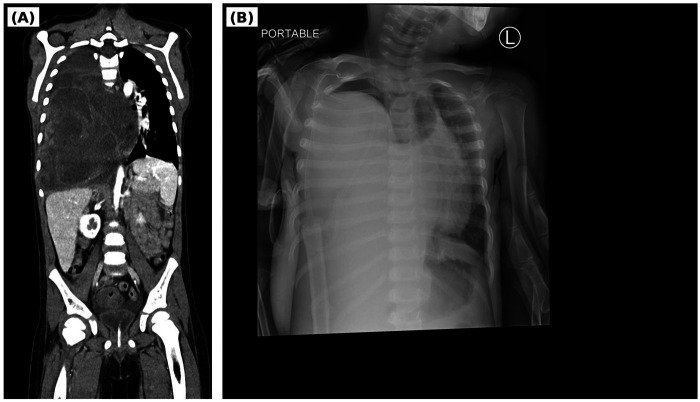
Representative imaging at presentation. (A) Coronal contrast-enhanced CT demonstrating a massive multiloculated predominantly cystic right hemithorax mass with marked mediastinal shift. (B) Portable chest radiograph demonstrating near-complete opacification of the right hemithorax with mass effect.
